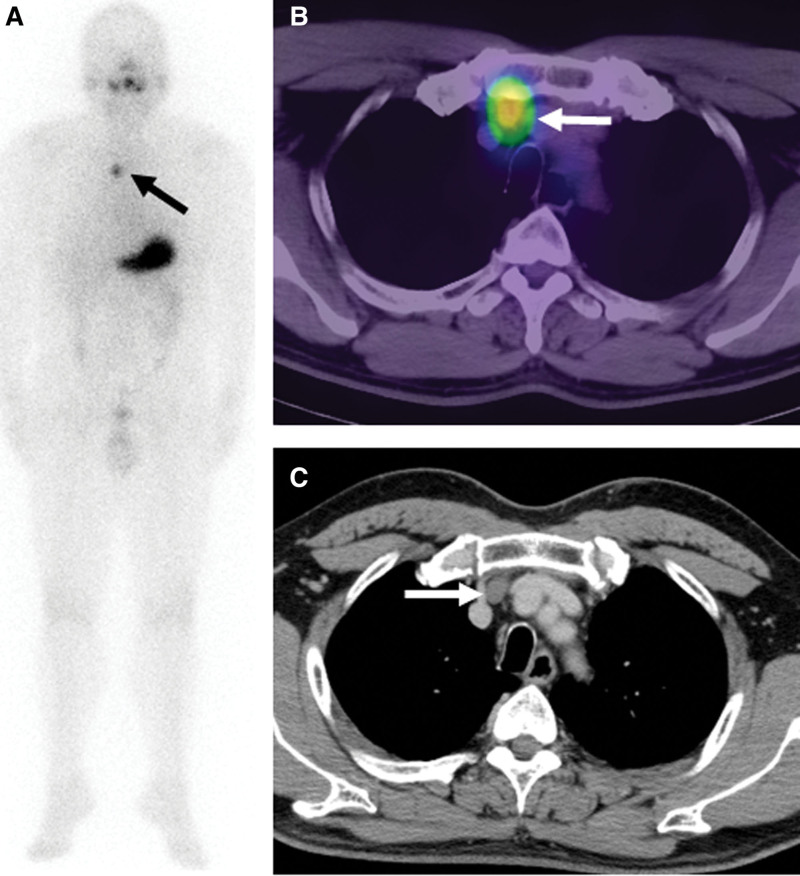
The positive uptake in the superior mediastinal nodule persisted on 131 WBS (A) and I-131 SPECT/CT (B) 3 days after the third therapeutic dose (arrows). Contrast-enhanced CT image (C) shows a nonclear contrast enhanced solid nodule (arrow).
